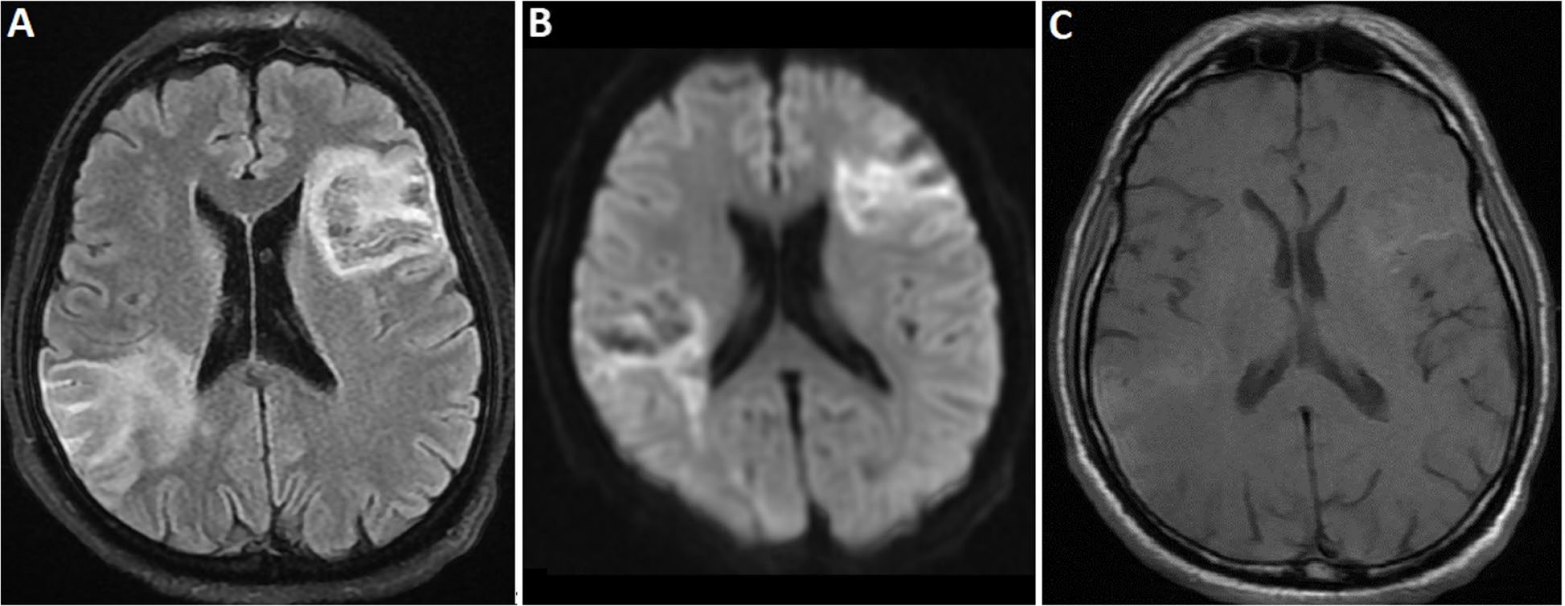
Brain MRI on day 8 showed subacute infarctions with restriction and mild hemorrhagic transformation in the right parietotemporal, left frontal lobe, and left centrum semiovale; A: Diffusion-weighted imaging (DWI); B: Fluid attenuated inversion recovery (FLAIR); C: T1-weighted
